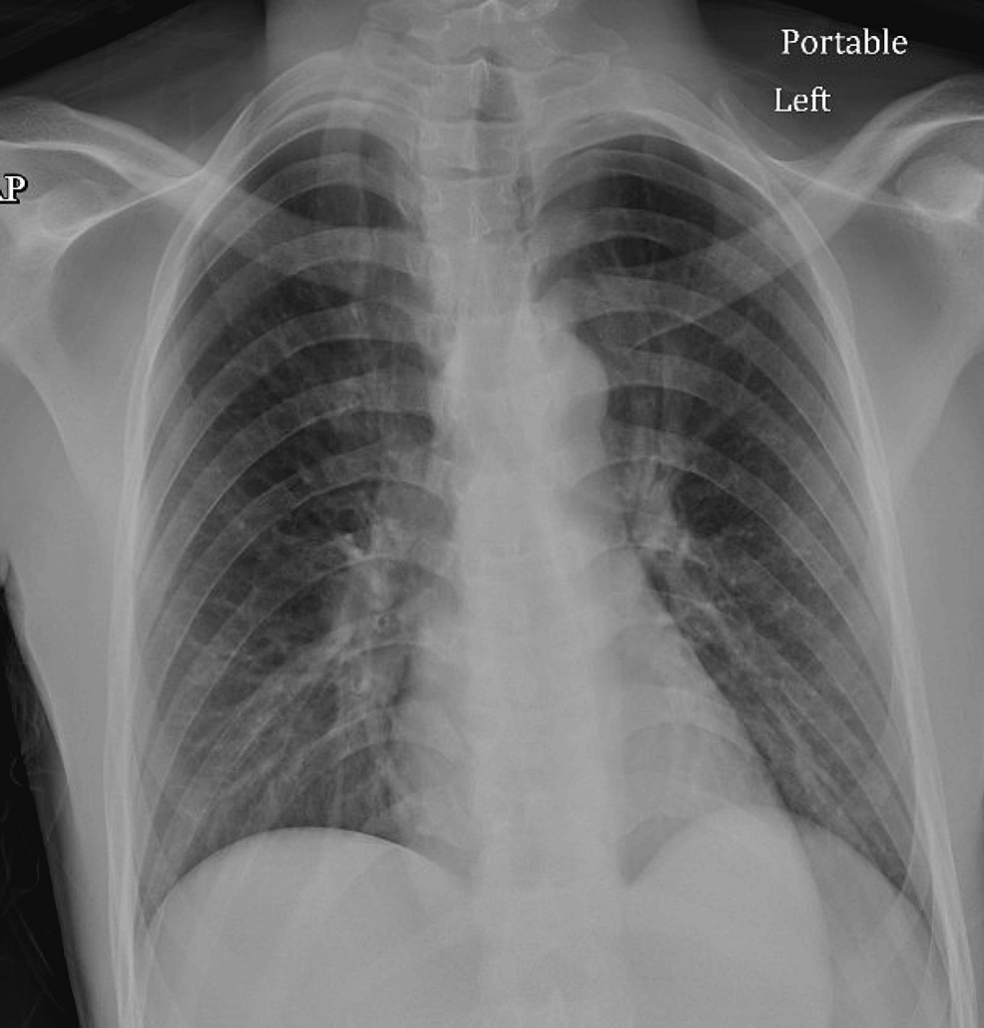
Portable chest x-ray showing no significant infiltrate or consolidation
